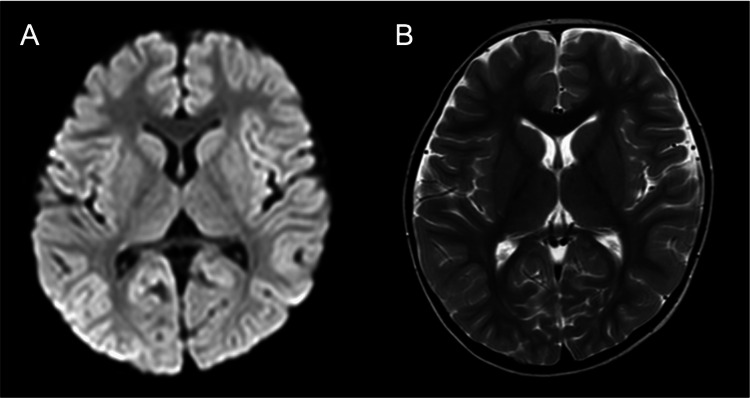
Head MRI There were no abnormal findings on the head MRI. (A) Diffusion-weighted image; (B) T2-weighted image
